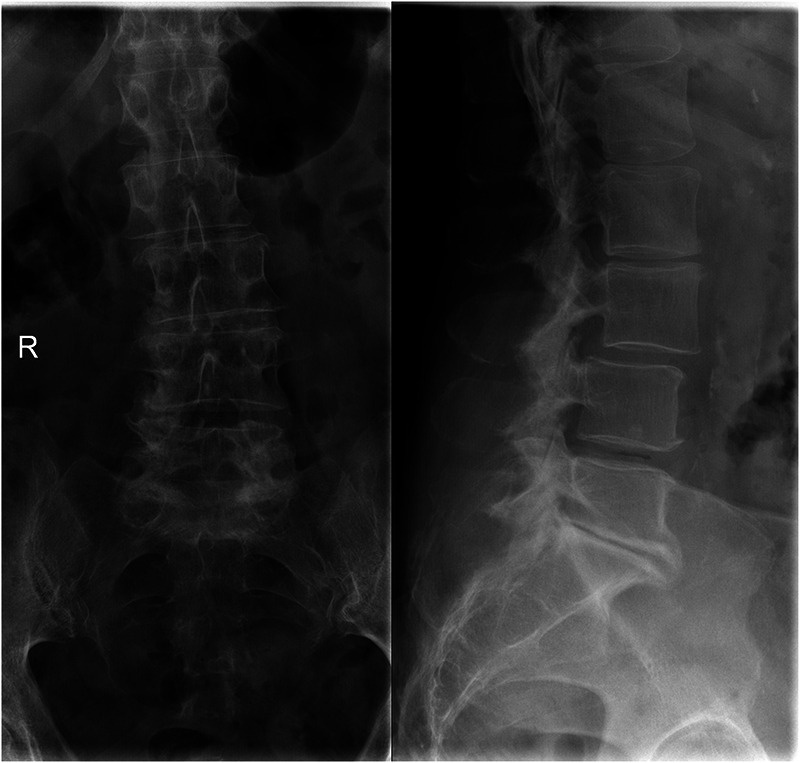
Preoperative standing x-ray prior to index surgery: anteroposterior view (left) and lateral view (right).
